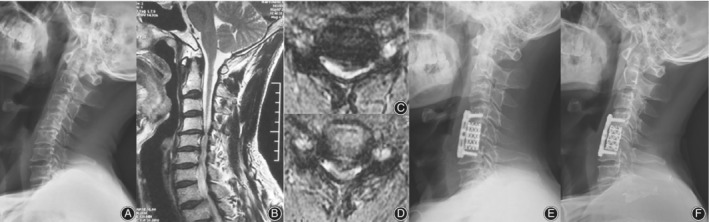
A 48‐year‐old male patient with proximal‐type CSA at the C_4/5_ and C_5/6_ levels. (A) The pre‐operative X‐rays of the cervical spine showing cervical hyperplasia bone hyperplasia, narrowed C_4/5_ and C_5/6_ intervertebral space. (B) Sagittal T2‐weighted magnetic resonance image showing cord compression at the C_4_‐C_6_ space. (C) Axial T2‐weighted magnetic resonance image at the C_4_‐C_5_ space showing both left ventral nerve root nerve root and spinal cord were compressed at the C_4‐5_ intervertebral level. (D) Axial T2‐ weighted magnetic resonance image at the C_5_‐C_6_ space showing narrowed segmental spinal canal and impingement against the left anterior horn nerve roots (white arrows). (E) Postoperative lateral X‐ray of the cervical spine showing that the internal fixation and the titanium cage were in appropriate position. (F) Follow‐up at 3 months, X‐ray of the cervical spine showing that the internal fixation is firm and maintained in appropriate position.
